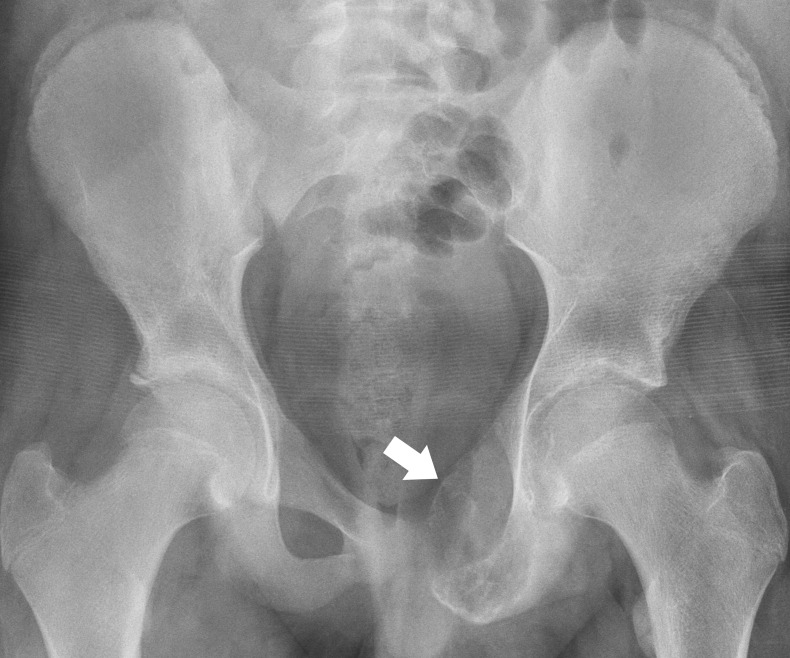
Radiograph of the Pelvis A 13-year-old male with an expansile bony lesion centered within the left pubic bone (white arrow) and involving the left superior pubic ramus, pubic body, and inferior pubic ramus. The lesion did not demonstrate gross cortical disruption, aggressive periosteal reaction, or an association with an overt soft tissue component.
